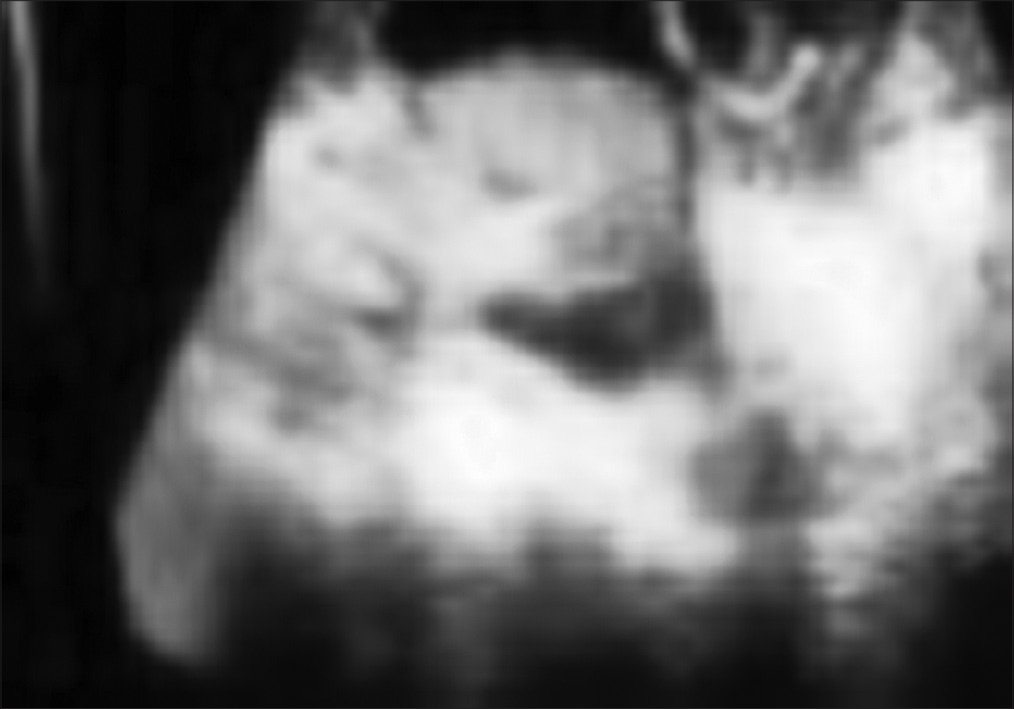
Ultrasonography showing intra-uterine pregnancy
